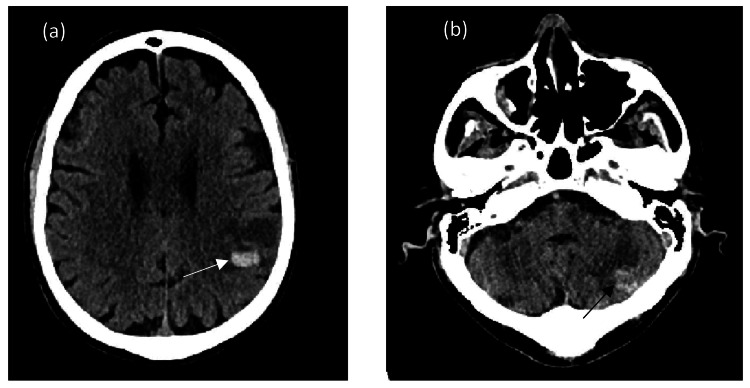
Plain axial CT demonstrating left posterior parietal (white solid arrow in (a)) and left cerebellar hemisphere (black arrow in (b)) with high attenuating haemorrhagic metastatic deposits and surrounding oedema
